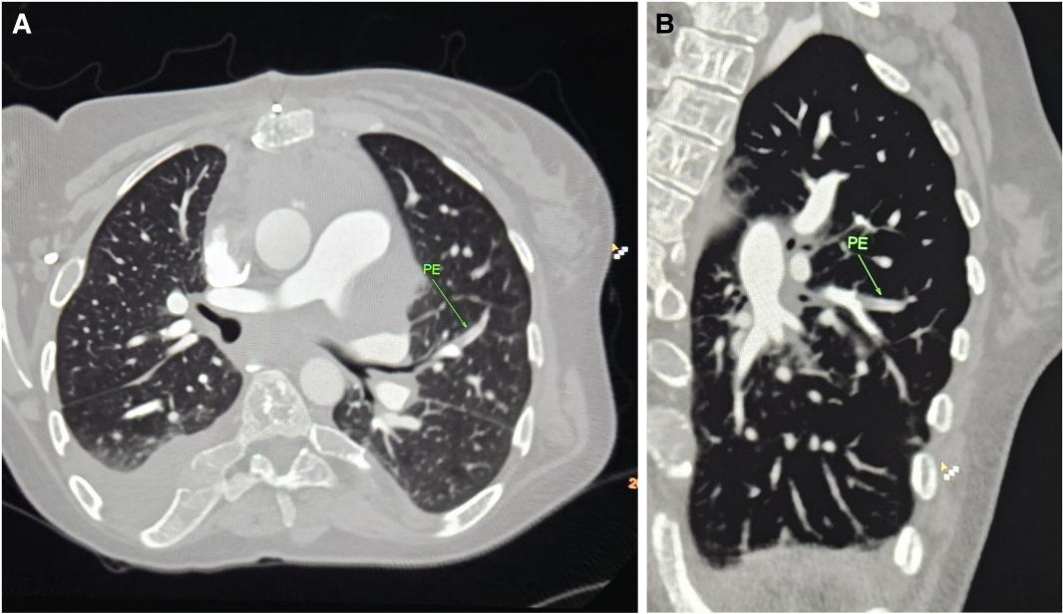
Chest computed tomography. PE, pulmonary embolus.
